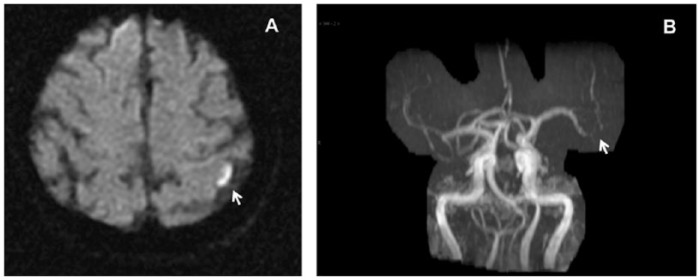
(A) Brain MRI showed a small acute left motor cortex infarct with spotty subcortical white matter ischemic lesions. The lesion in the left precentral gyrus correlated with the history of right upper extremity weakness. (B) MRA suggested the presence of an embolic stroke by identifying irregular segmental narrowing of the angular branch of the left middle cerebral artery, of concern for recanalization stenosis with either spontaneous fragmentation or lysed thrombus fragmentation.
